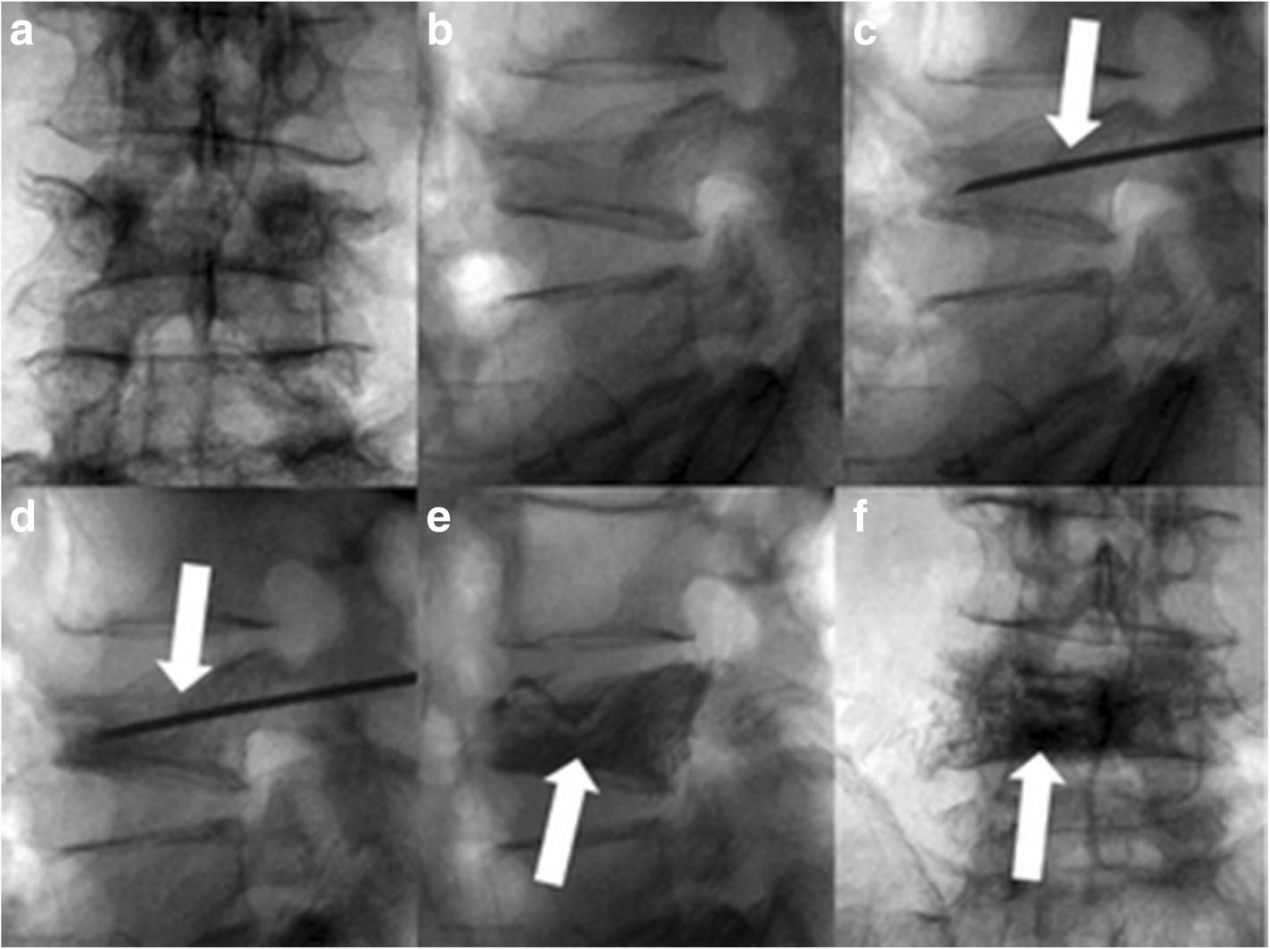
Same case as shown in Fig. 1. Elastoplasty procedure: injection of VK100 in the fractured body (L4) using the transpedicular approach under fluoroscopic guidance. Preprocedural control x-rays; anteroposterior (a) and sagittal (b) view; fluoroscopy during transpedicular needle insertion (arrow) showing correct placement of the needle into the vertebral body (c, d); postprocedural control x-rays showing the good filling of L4 body (arrow, e, f)
